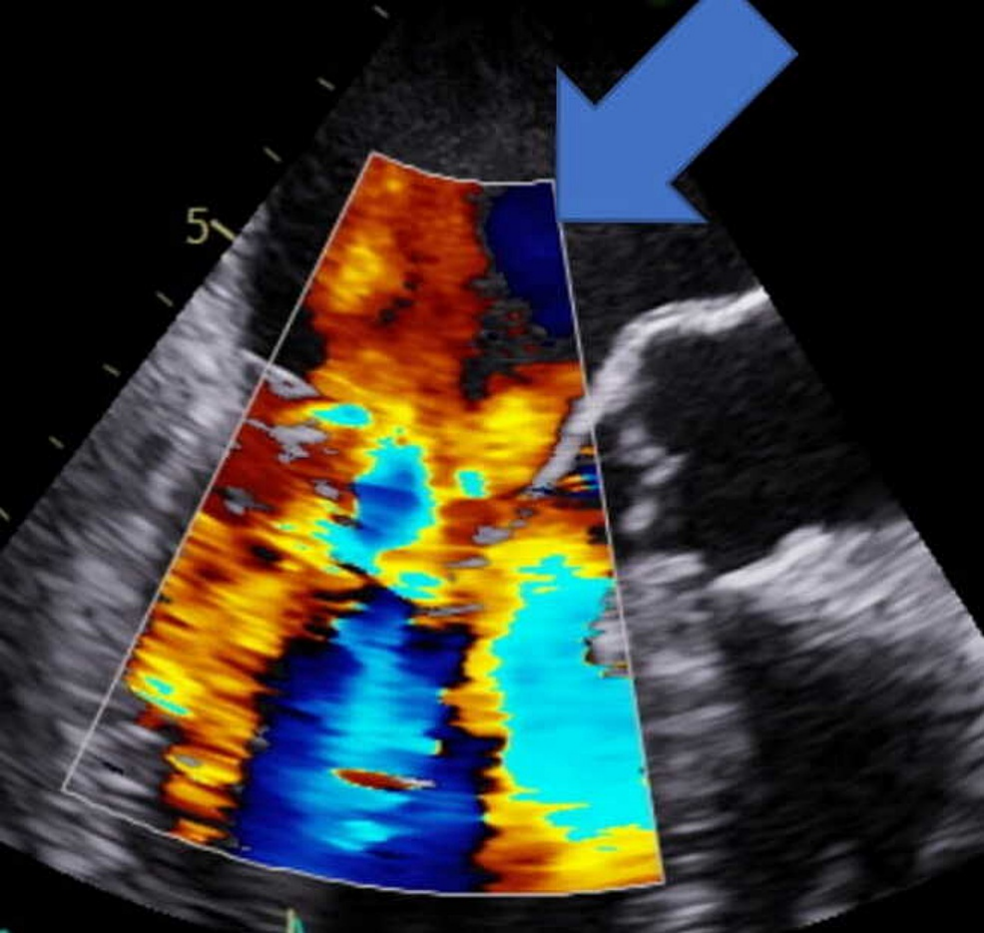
Transoesophageal echocardiogram showing severe mitral valve regurgitation
